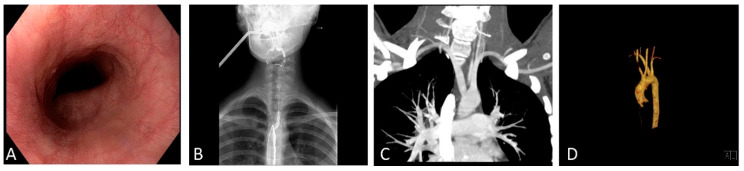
(A) Oesophagogastroduodenoscopy showing an image of an ab extrinseco compression of the mid-cervical oesophagus. (B) Upper gastrointestinal tract radiography confirming an oesophageal impression above the arch of the aorta. (C) Computed tomography angiography and (D) three-dimensional visualization, which detect the presence of a lusoria artery originating from the medial margin of the descending aorta and crossing the trachea and oesophagus posteriorly to the distal third, resulting in a tortuous appearance.
